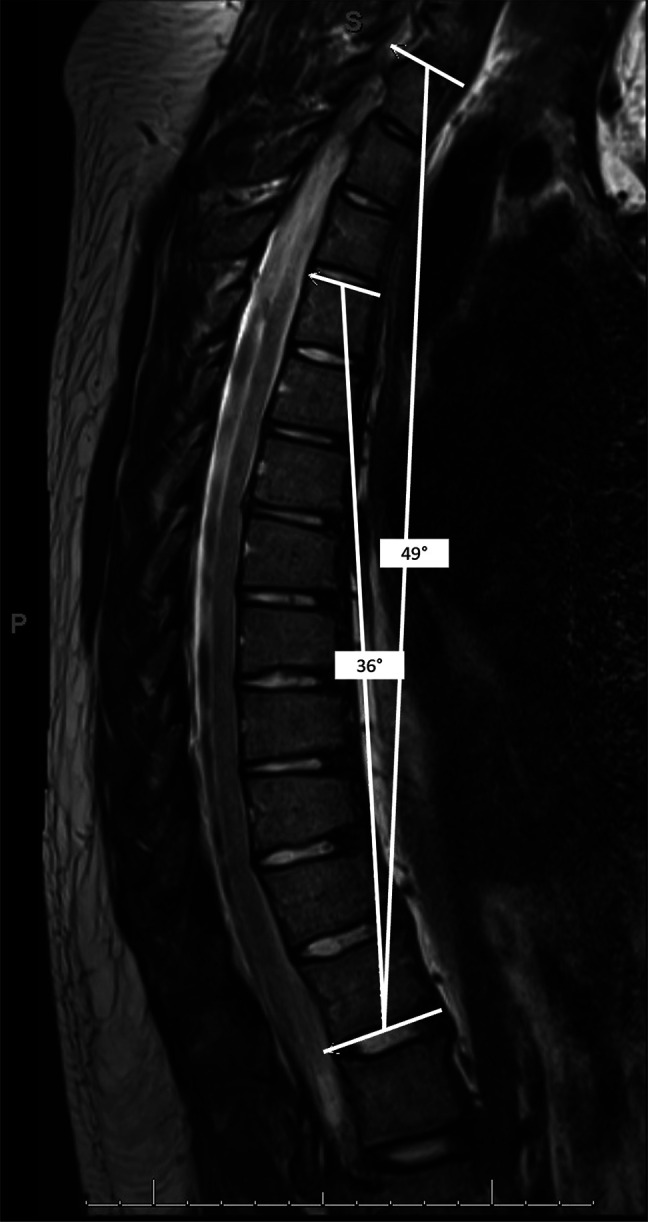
Image showing decreased T1 to T12 angle and T4 to T12 thoracic kyphosis measurements on the T2-weighted thoracic spine magnetic resonance imaging compared with standing measurements demonstrated the flexibility of the patient's curve.
